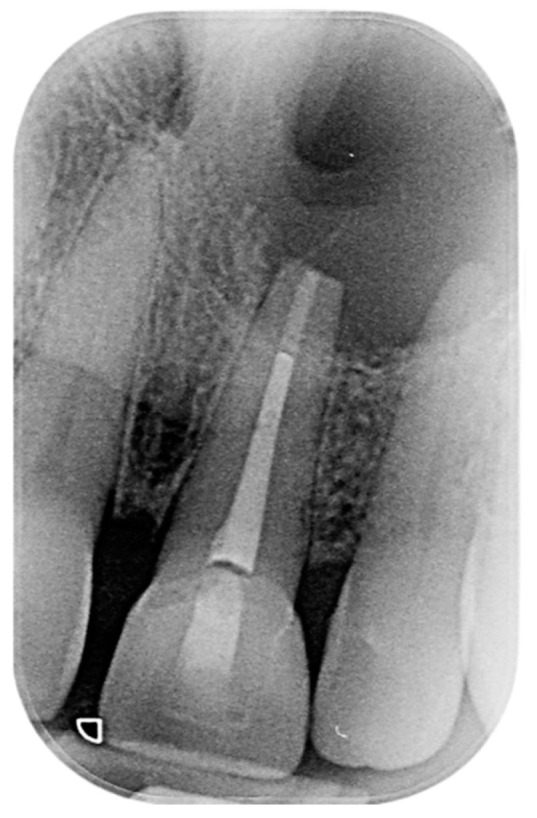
Post-surgery intraoral periapical radiograph of the upper left central and lateral incisors.
